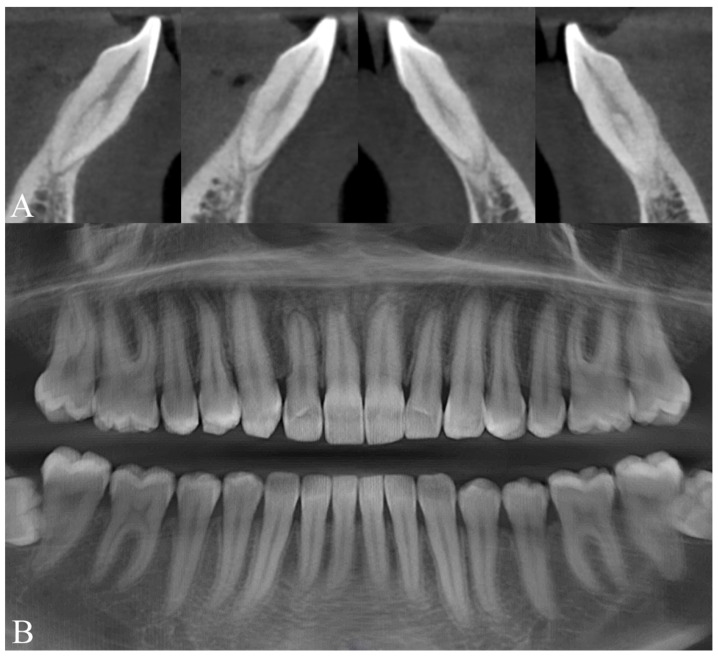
Initial radiographic records of Clinical Case 2. (A) CBCT sections of the mandibular anterior region. (B) Panoramic radiograph reconstructed from the CBCT.
